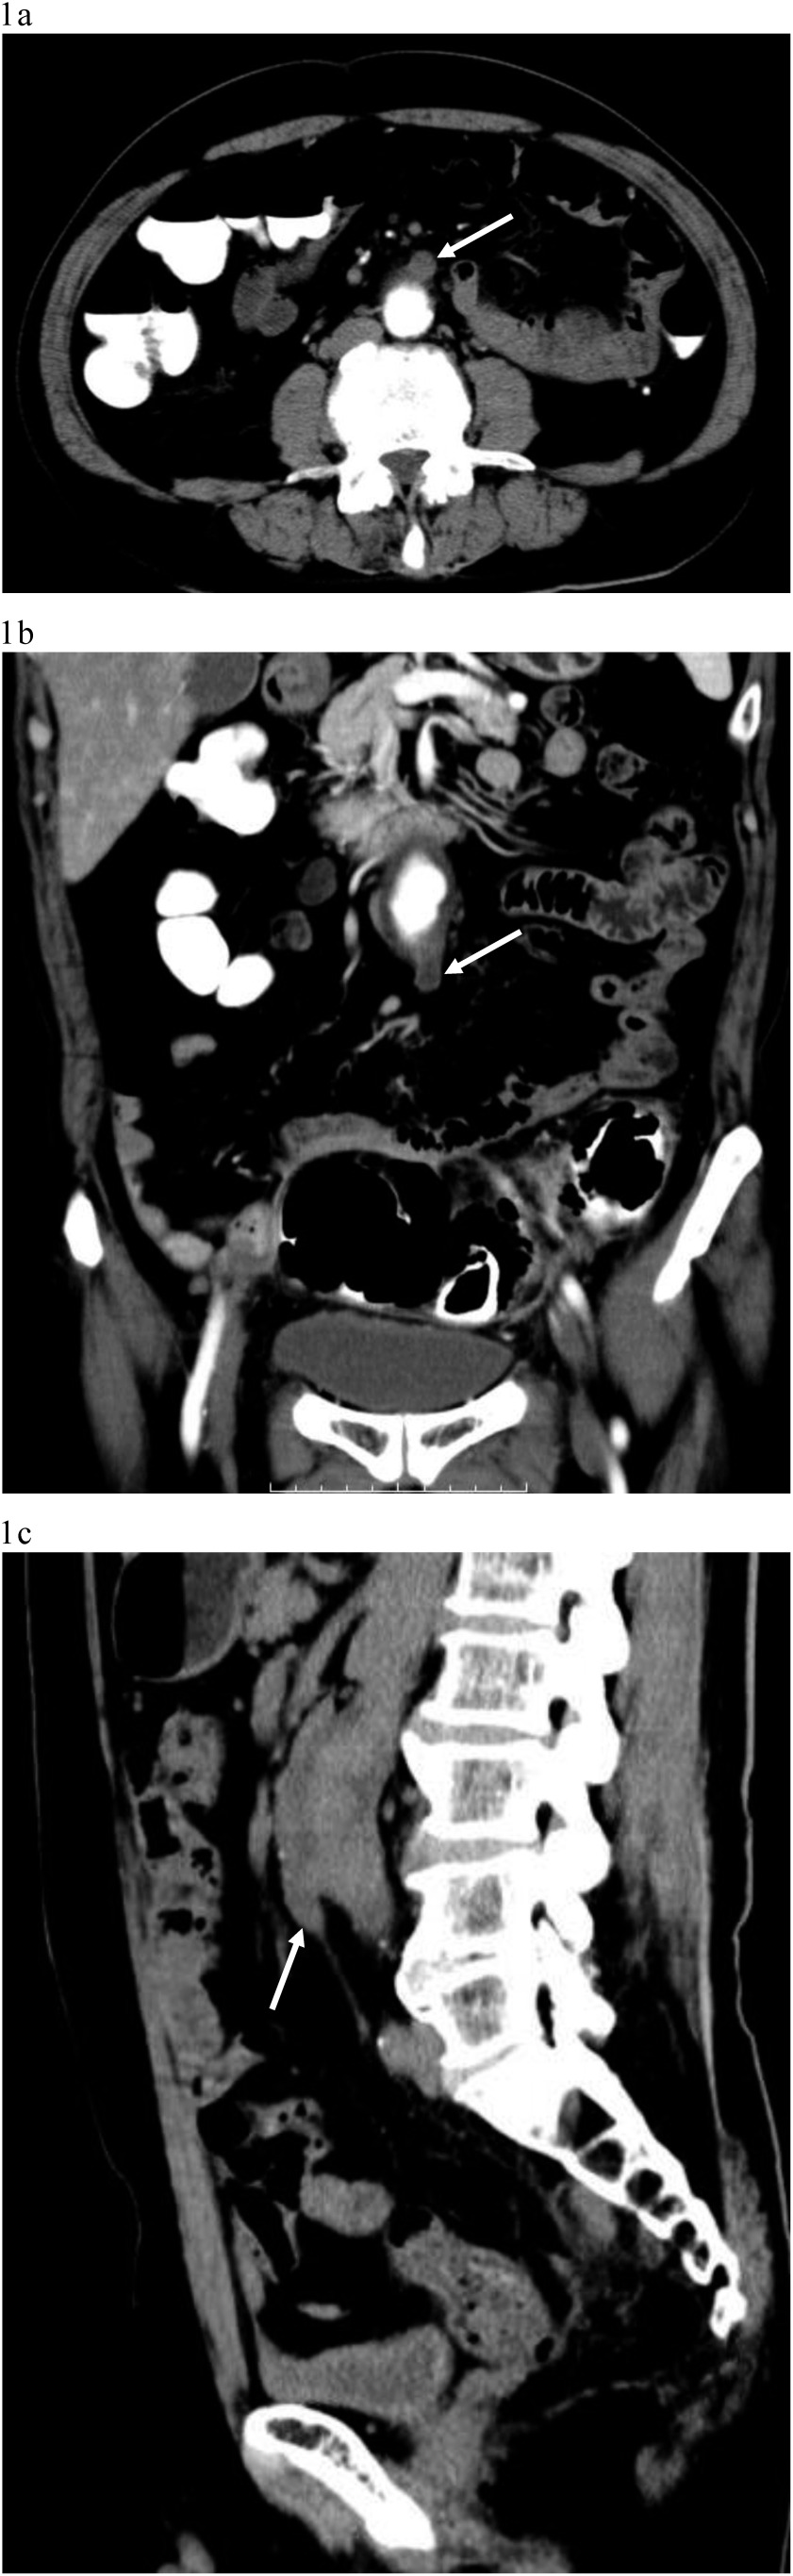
Fig. 1 Contrast-enhanced computed tomography at 7 years before endovascular aneurysm repair. (a), (b), and (c) show axial, coronal, and sagittal views, respectively. The inferior mesenteric artery (white arrow) is already aneurysmal and thrombosed.
